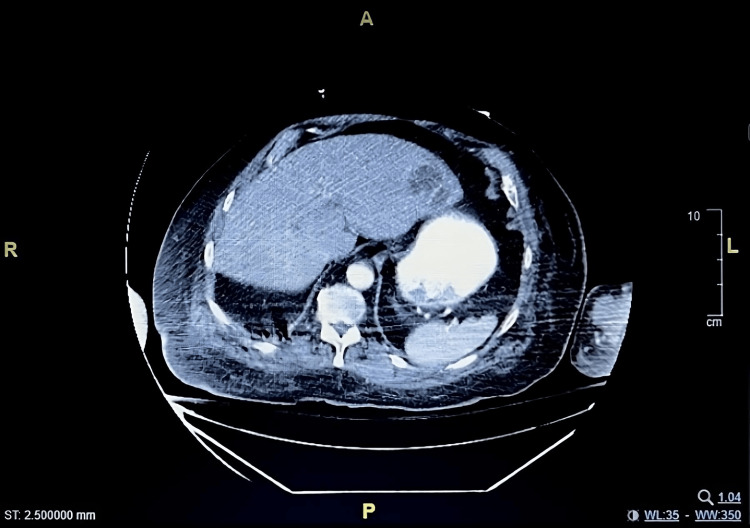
Contrast-enhanced computed tomography in axial view shows a multi-septated cystic structure in the left lobe of the liver measuring 4.5 x 2.7 cm.
